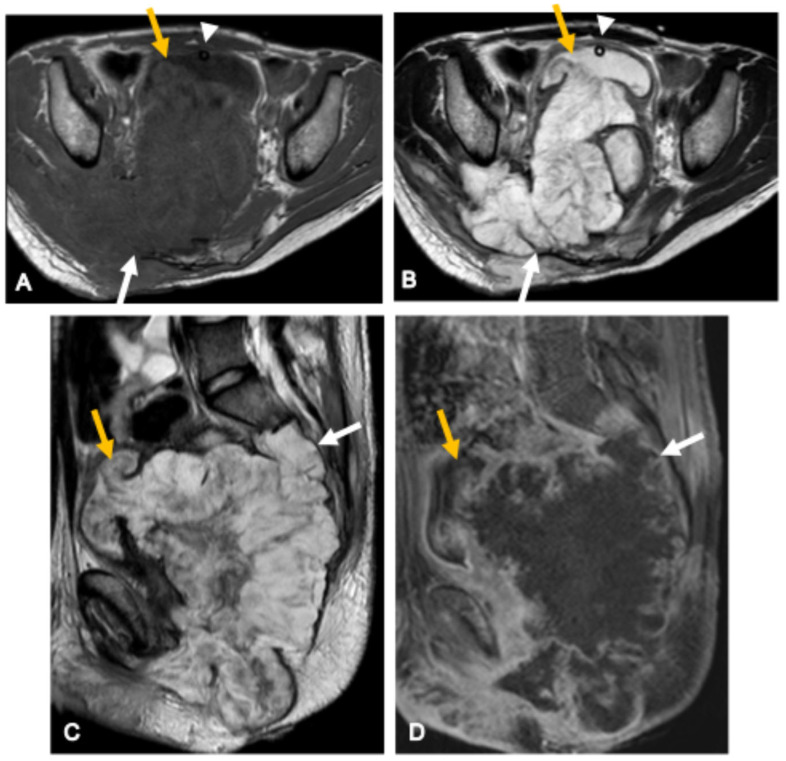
Intestinal adenocarcinoma with sacral invasion in a 50-year-old man. Axial T1-weighted (A) and T2-weighted (B) images, as well as sagittal T2-weighted (C) and contrast-enhanced fat-suppressed T1-weighted (D) images, demonstrate a large expansile pelvic mass invading the sacrum (white arrows) and urinary bladder (yellow arrows). The lesion exhibits predominantly high T2 signal intensity, peripheral enhancement after contrast administration, and extensive central necrosis. A Foley catheter is visible within the bladder (arrowheads).
